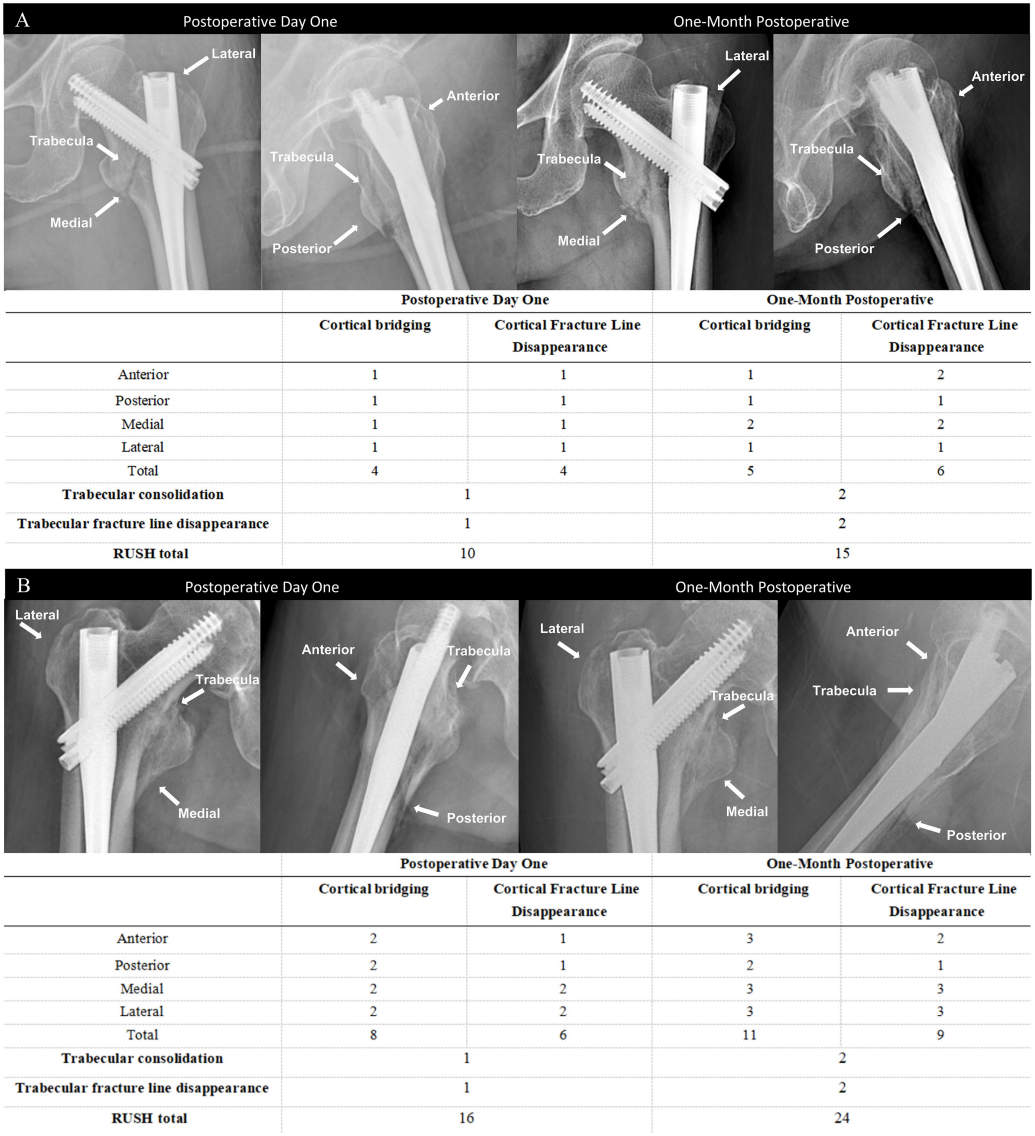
Anteroposterior and lateral radiographs obtained at postoperative day 1 and at 1 month in two patients with relatively slow (A) and fast (B) fracture healing, along with the corresponding RUSH scores.
